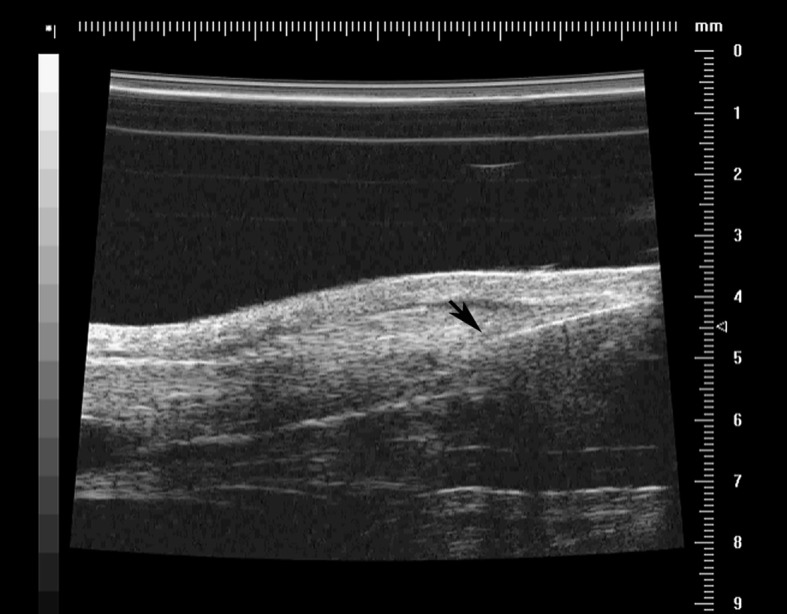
Ultrasound (US)-guided intratendinous injection. The needle (arrow) is clearly visualized with US guidance. The image of the tendon tissue expansion confirms the effect of the high-volume intratendinous injection.
